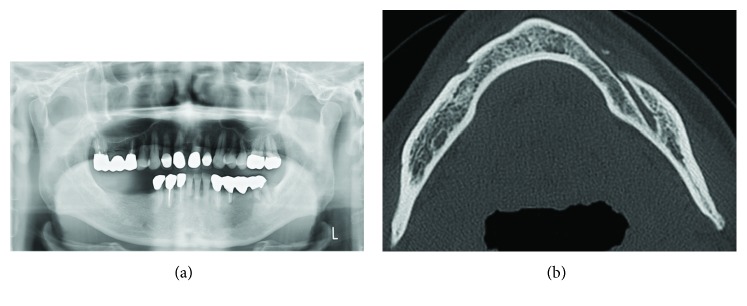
Images of the jaw at 96 months after surgery. The panoramic dental radiograph (a) and computed tomography image (b) show no signs of recurrence.
